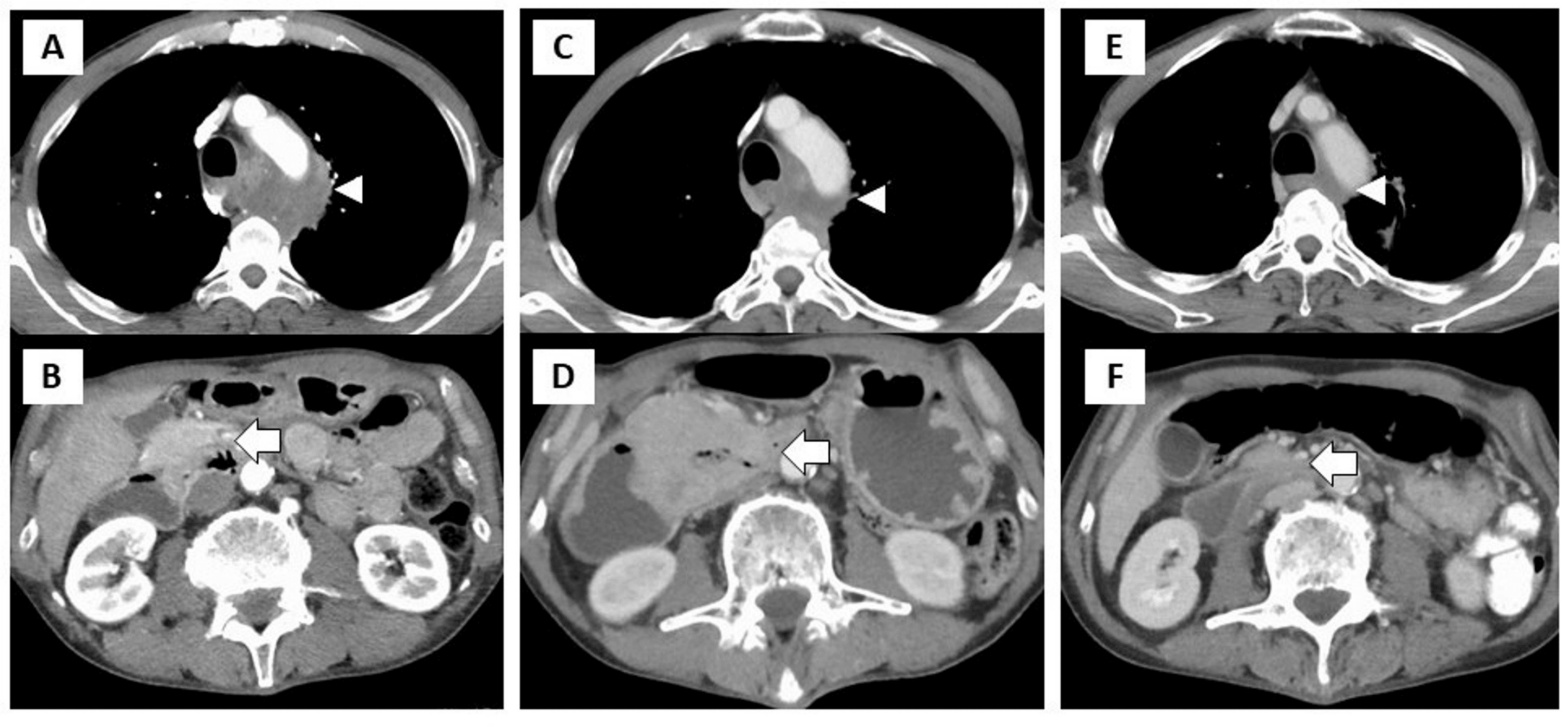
Contrast-enhanced CT findings of chest and abdomen. CT at diagnosis showed a metastatic tumor in the mediastinal lymph nodes (arrowhead) (A) and small intestine (arrow) (B). CT at readmission for gastrointestinal symptoms showed shrinkage of the mediastinal tumor (C) and marked enlargement of the small intestinal tumor (D). Improvement of both mediastinal and intestinal tumors was observed nine months after pembrolizumab treatment (E, F). CT, computed tomography
